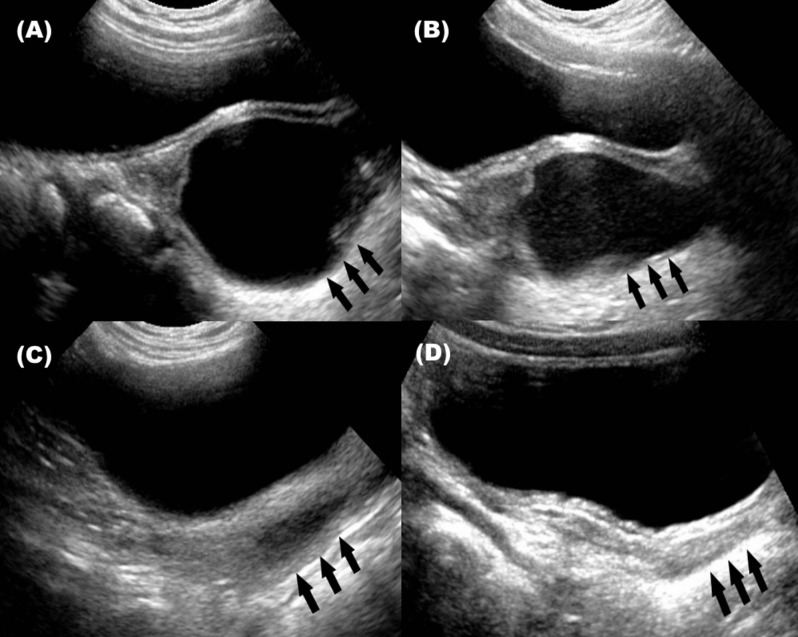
Serial ultrasonography of six-year-old girl who demonstrated complete resolution of hemivaginal distention. (A) Ultrasonography of initial hemivaginal distention performed at 10 months (B),(C) Ultrasonography performed at 16 months and 24 months showing partial resolution of hemivaginal distention (C) Ultrasonography performed at thirty months showing complete resolution. *Arrow head: obstructed hemivagina
